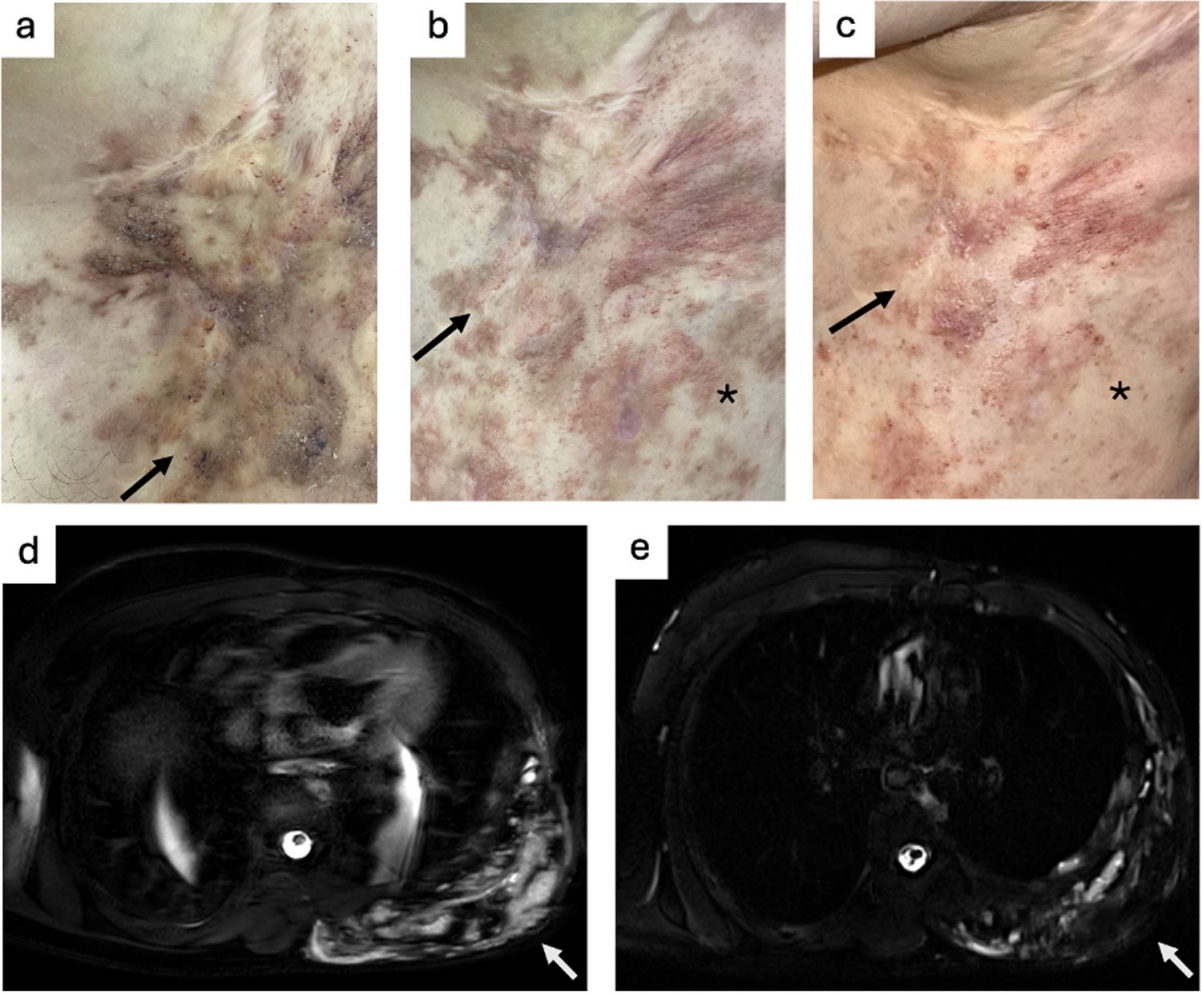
23-year-old male patient with microcystic lymphatic malformations (LMs) of the left treated by two sessions of Bleomycin-electrosclerotherapy (BEST) a Clinical photograph before BEST treatment; note the extensive skin involvement including numerous small, translucent and red–purple vesicles, hyperkeratosis with verrucous texture due to chronic lymphatic changes and the mix of red and brownish discolouration following haemosiderin deposition (arrow). Extensive scarring following prior surgical treatment. b Clinical photograph 8 weeks after the first BEST session presenting already regressing chronic skin changes (arrow) alongside prominent postprocedural skin discolourations at electrode insertion sites (asterisk). c Significantly improved LM-associated chronic skin changes after second BEST session (arrow) as well as almost complete fading of postprocedural skin discolouration (asterisk). d T2-weighted magnetic resonance imaging (MRI) sequence showing the extensive microcystic LM of the left trunk before treatment (arrow). e) Follow-up MRI 6 months after second BEST session with significantly regressed findings (arrow), corresponding to the clinical improvement
